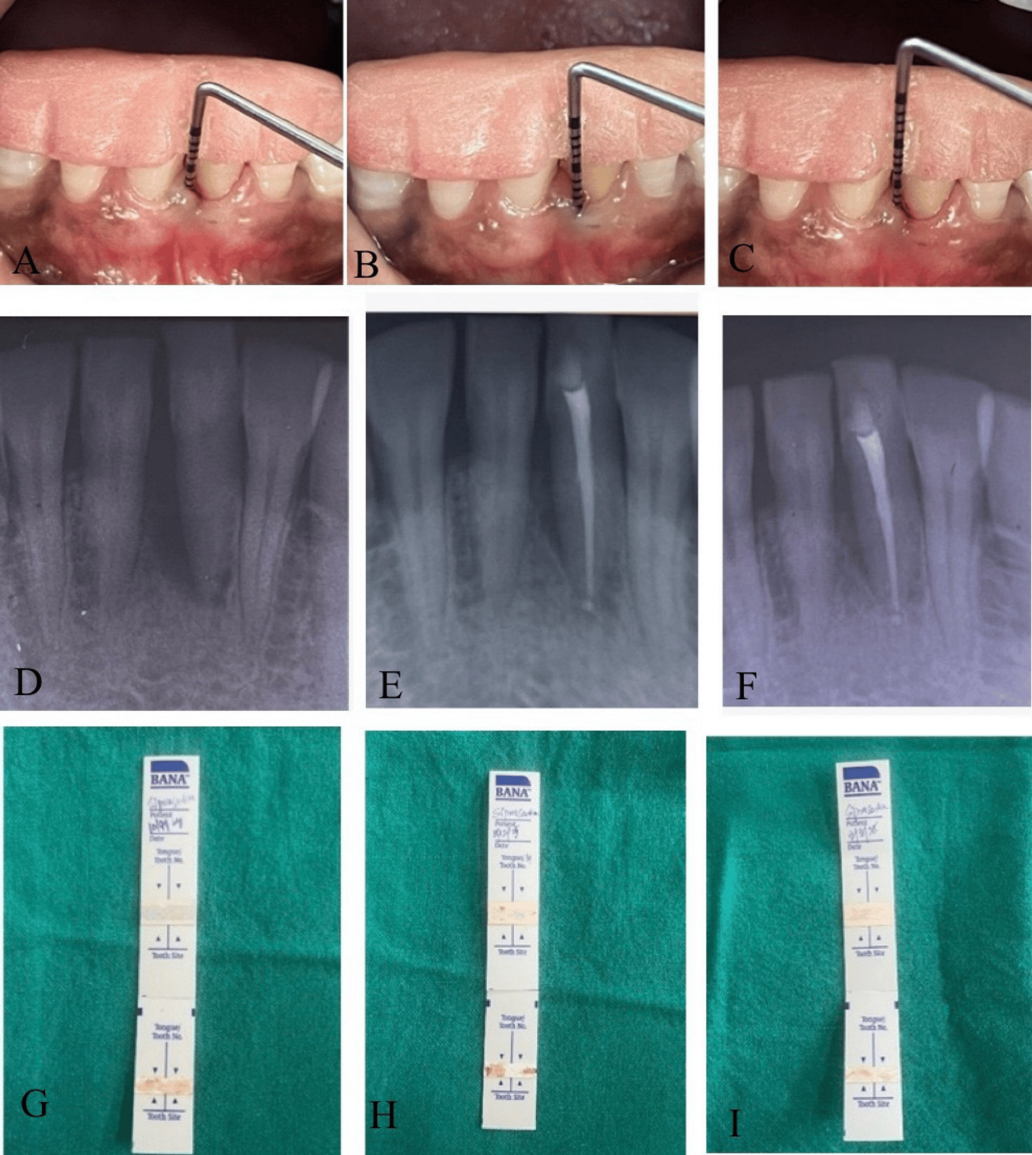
Sequential clinical, radiographic, and enzymatic evaluation of the treatment site at baseline (A, D, G), three-month follow-up (B, E, H), and six-month follow-up (C, F, I) demonstrating progressive reduction in periodontal pocket depth, periapical healing following endodontic and periodontal therapy, and a corresponding decrease in the "red complex" bacterial activity as shown by BANA test strip transitions from positive (dark blue) to negative (salmon) BANA: N-benzoyl-DL-arginine-2-naphthylamide.
